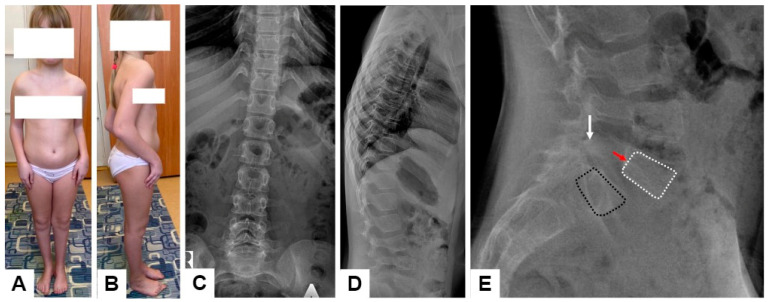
Appearance of the patient F14-P15 with AG-like phenotype (A,B). X-rays of the spine in frontal and lateral projections (C,D). Lateral radiographs of the lumbosacral region of the patient with spondylolytic spondylolisthesis: spondylolysis of the L5 vertebral arch (white arrows) and anterior displacement (red arrows) of the L5 bodies (white dotted lines) in relation to the S1 (black dotted lines) (E).
